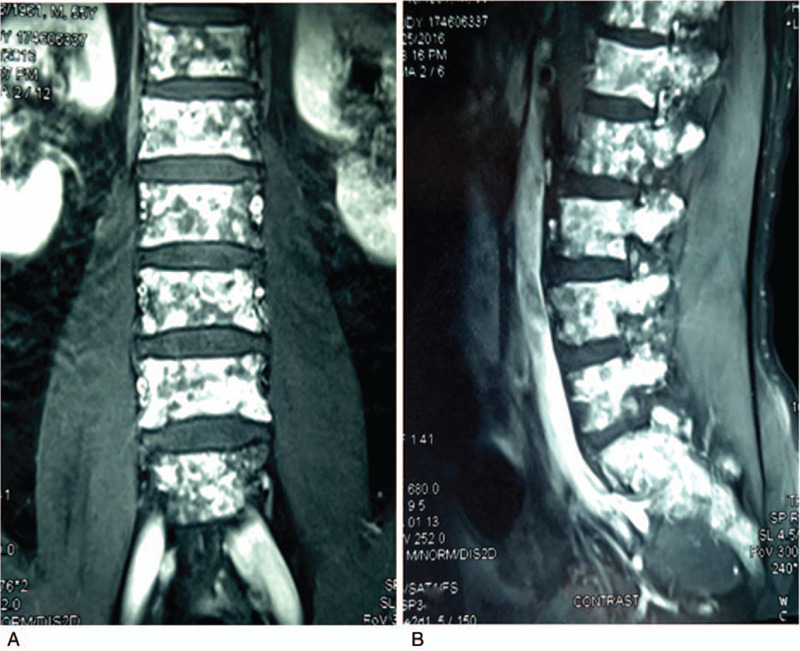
Lumbar MRI exhibiting an extensive bone lytic lesion of the the the whole spine. No tumor in other body soft tissues or organs was identified (2A at coronal plane, 2B at sagittal plane).
